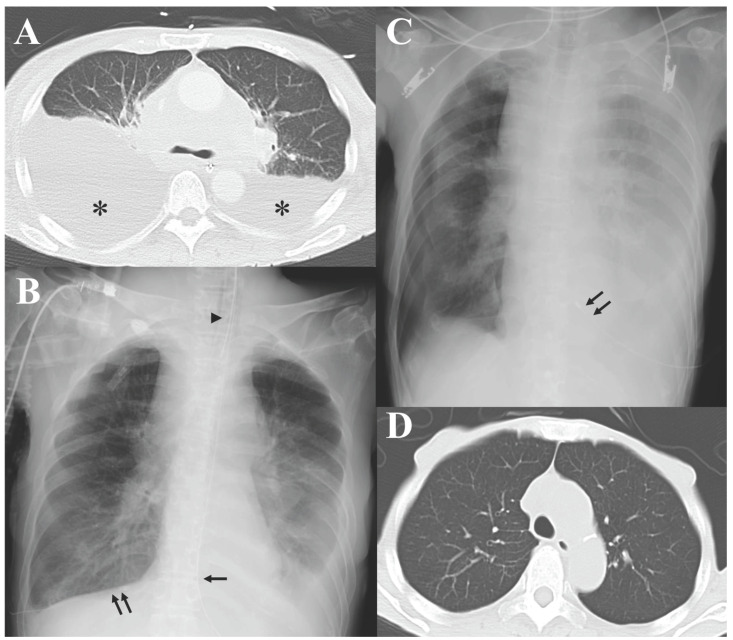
Radiological course of bilateral pleural effusions. (A): Chest computed tomography (CT) obtained immediately after endotracheal intubation, showing bilateral pleural effusions (asterisks), more prominent on the right side. (B): Chest X-ray during right-sided continuous thoracic drainage, showing a decrease in the right pleural effusion and persistent left pleural effusion; the arrowhead indicates the endotracheal tube, the arrow indicates the nasogastric tube, and the double arrows indicate the right chest tube. (C): Chest X-ray obtained after extubation and removal of the right chest tube, demonstrating progression of the left pleural effusion and the newly inserted left chest tube (double arrows). (D): Chest CT after immunochemotherapy and removal of both chest tubes, showing resolution of the bilateral pleural effusions without re-accumulation.
